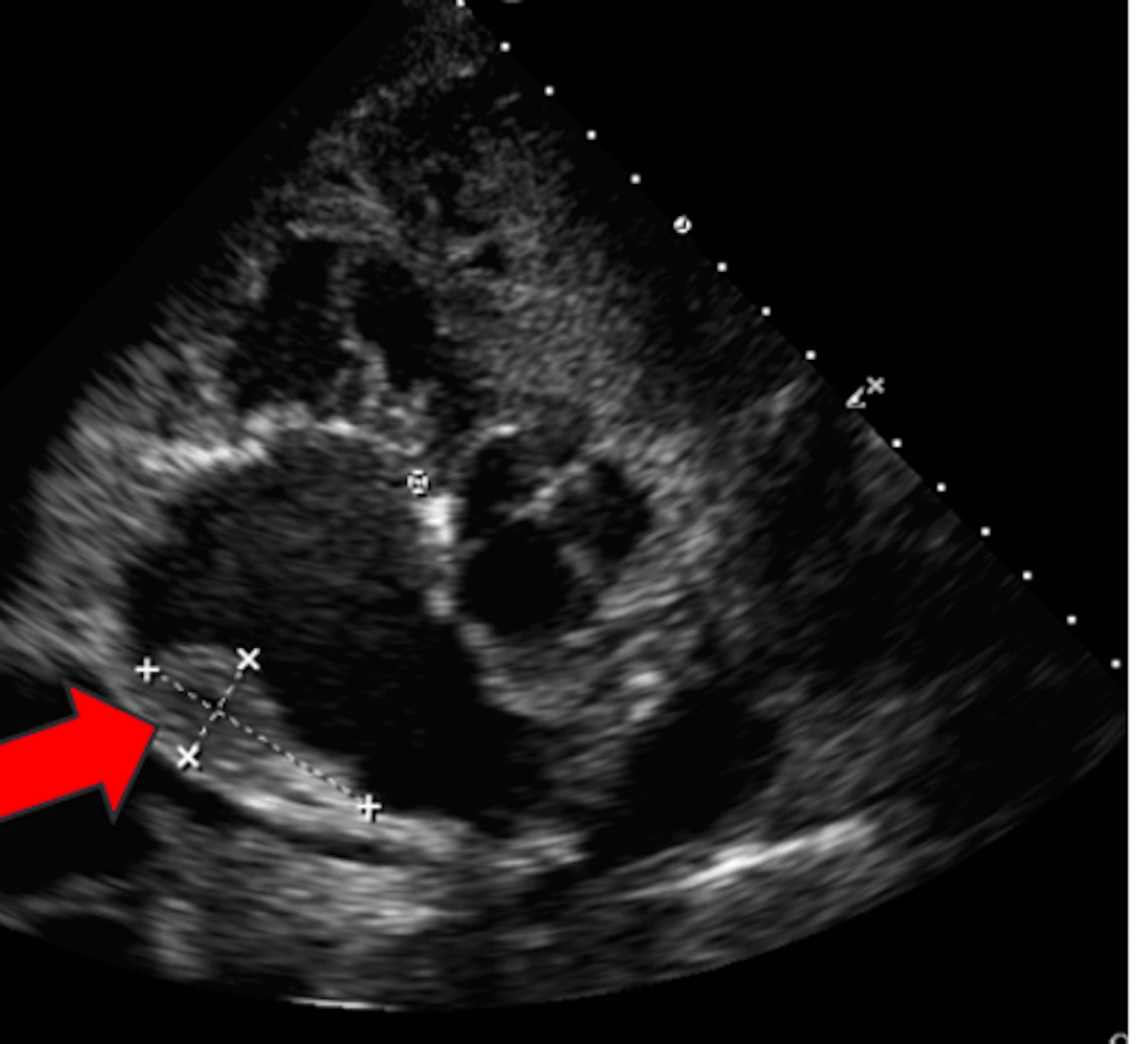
Parasternal short axis view showing a right atrial thrombus A non-mobile echogenic mass is visualized in the right atrium, consistent with thrombus formation. This finding may reflect severe atrial stasis secondary to restrictive cardiomyopathy in the context of cardiac amyloidosis.
